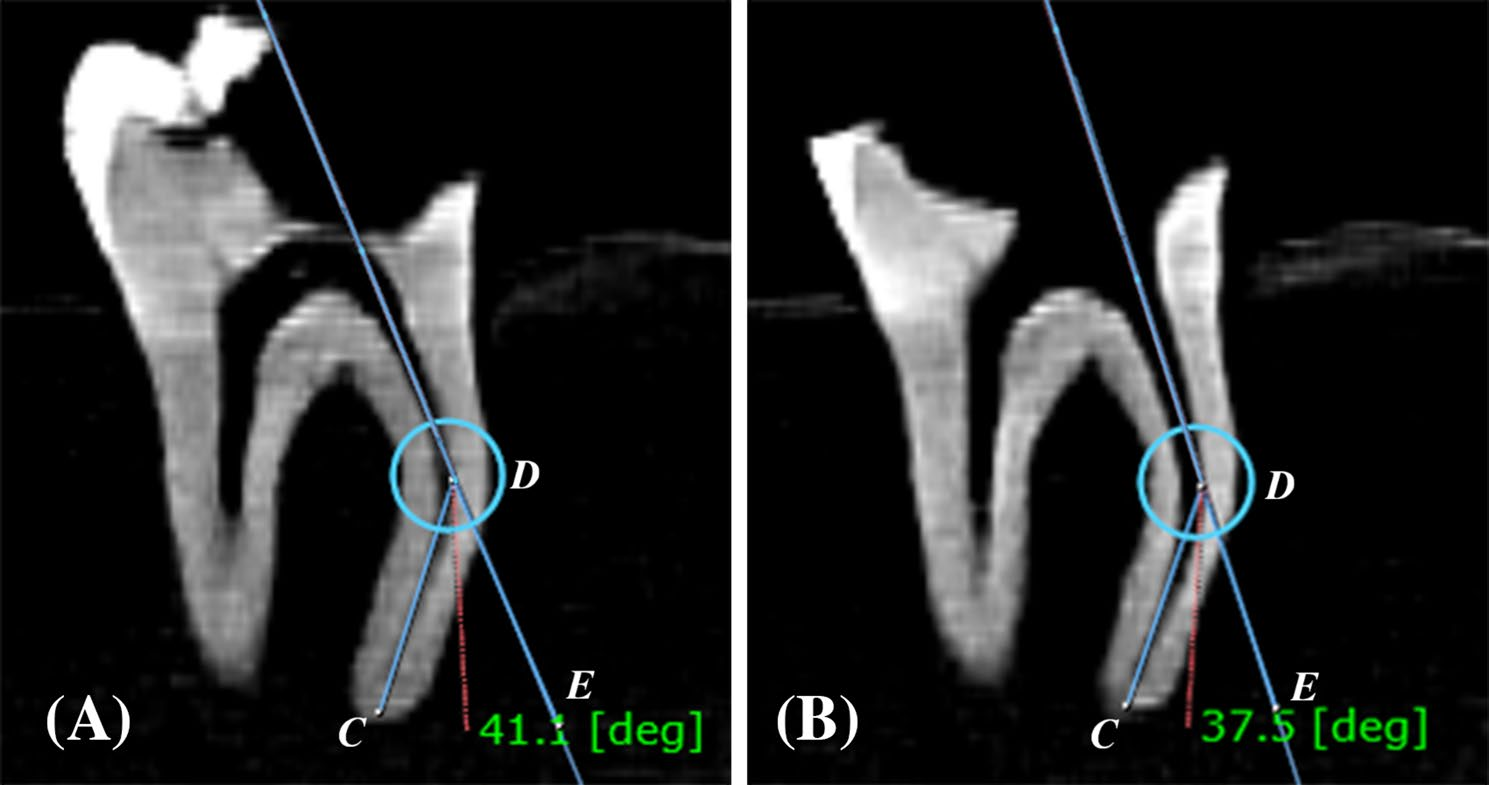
Representing CBCT sagittal view to measure Schneider angle of curvature CDE. A preoperative angle of curvature. B postoperative angle of curvature
